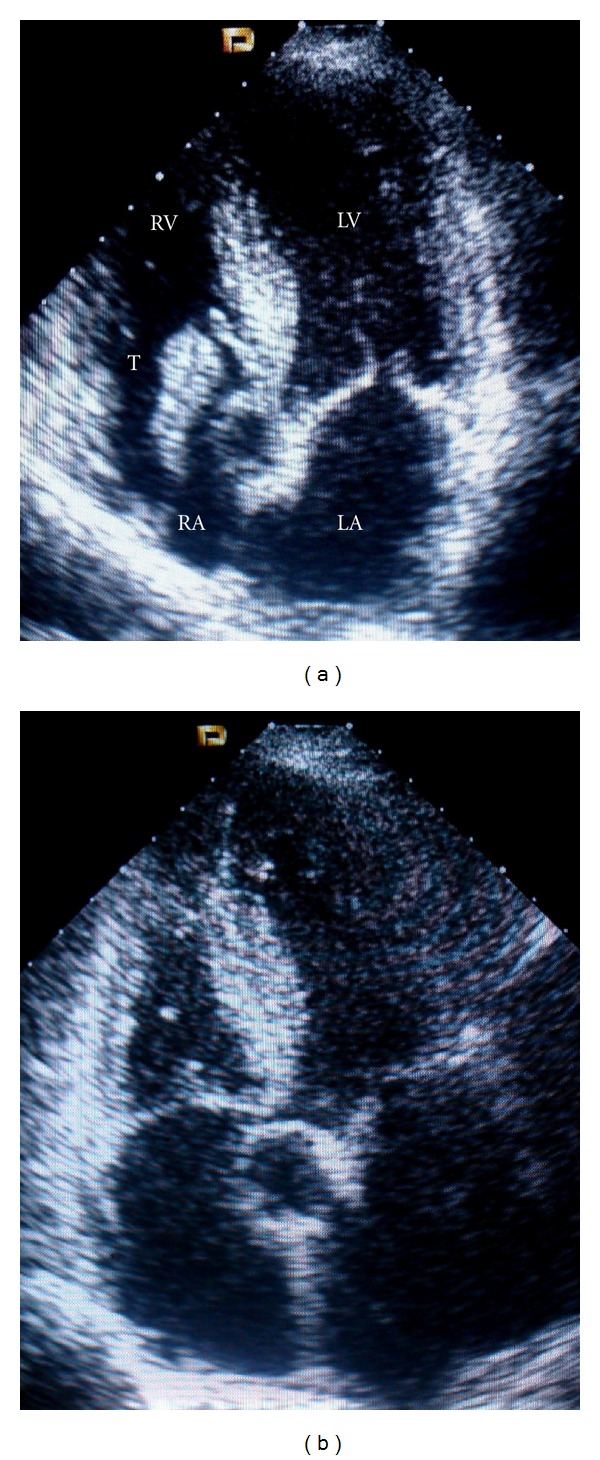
(a) Transthoracic apical five-chamber view showing the thrombus with thin stalk prolapsing through the tricuspid valve. (b) Same view after oral anticoagulation therapy. Complete dissolution of the thrombus. LA, left atrium; LV, left ventricle; RA, right atrium; RV, right ventricle; T, thrombus.
